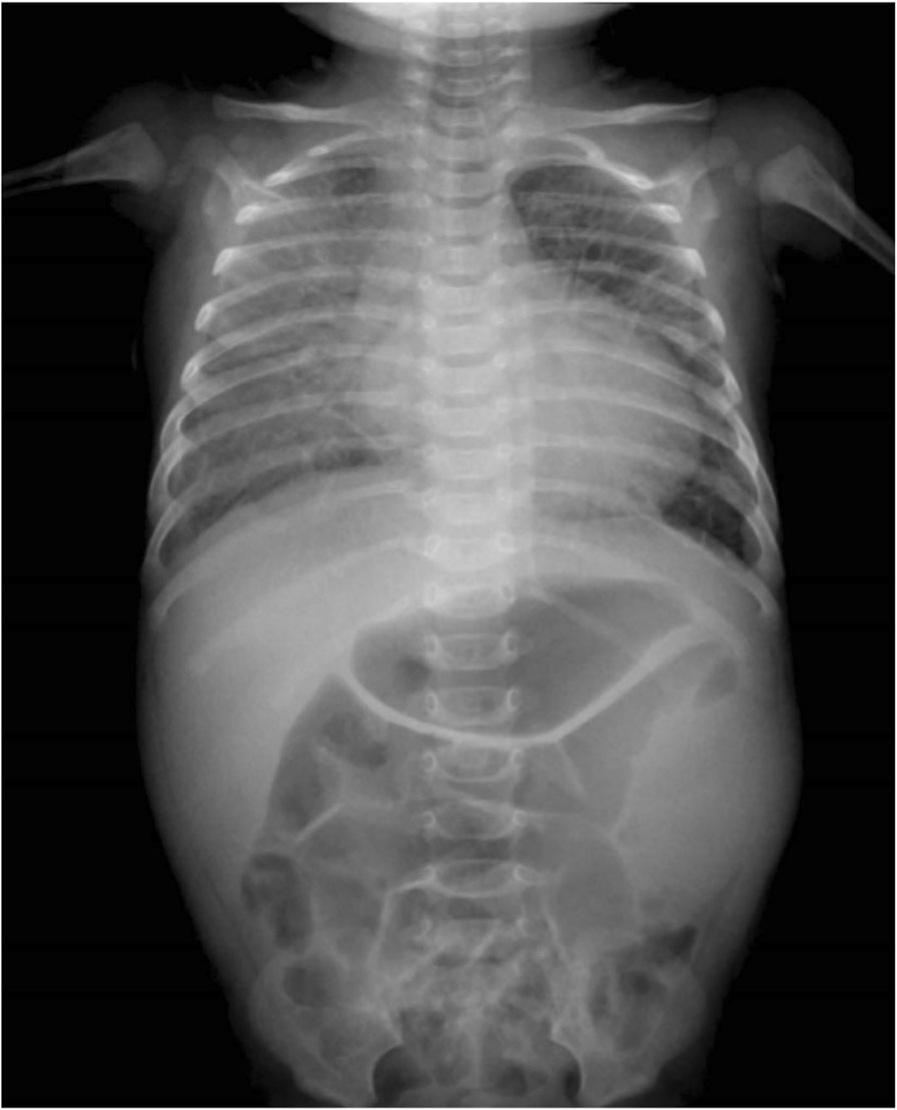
A chest X-ray showed cardiomegaly and pulmonary edema on admission to the ICU
